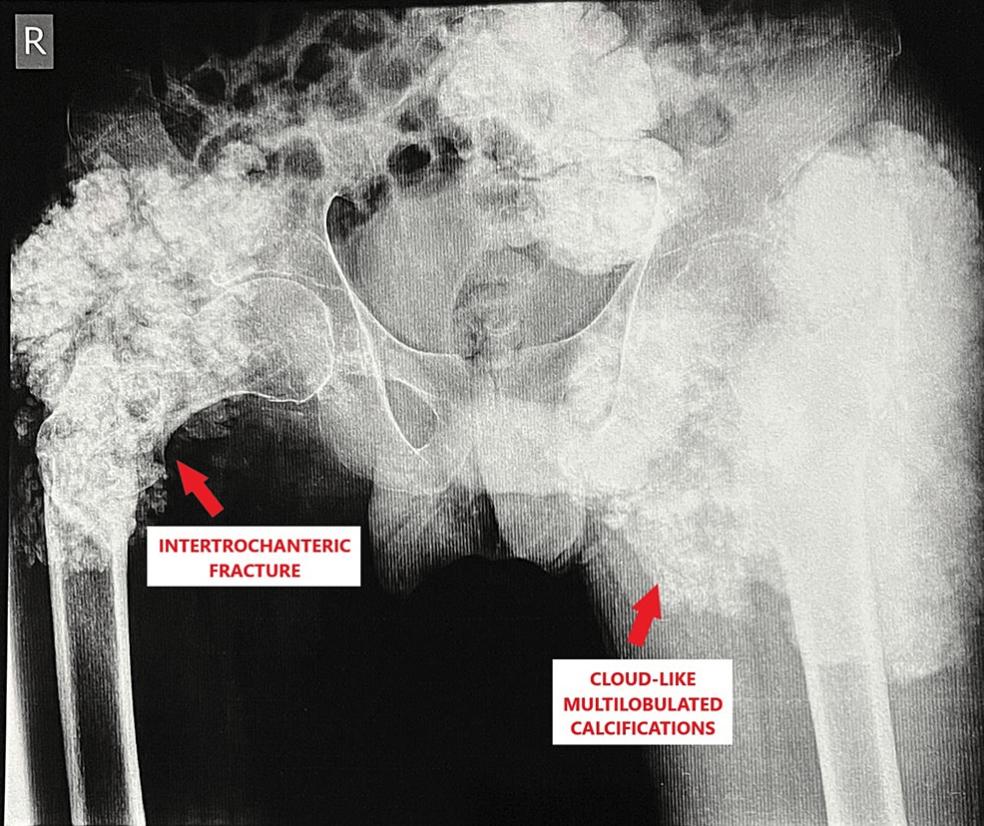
Plain radiograph showing pelvis and both hip joints.
